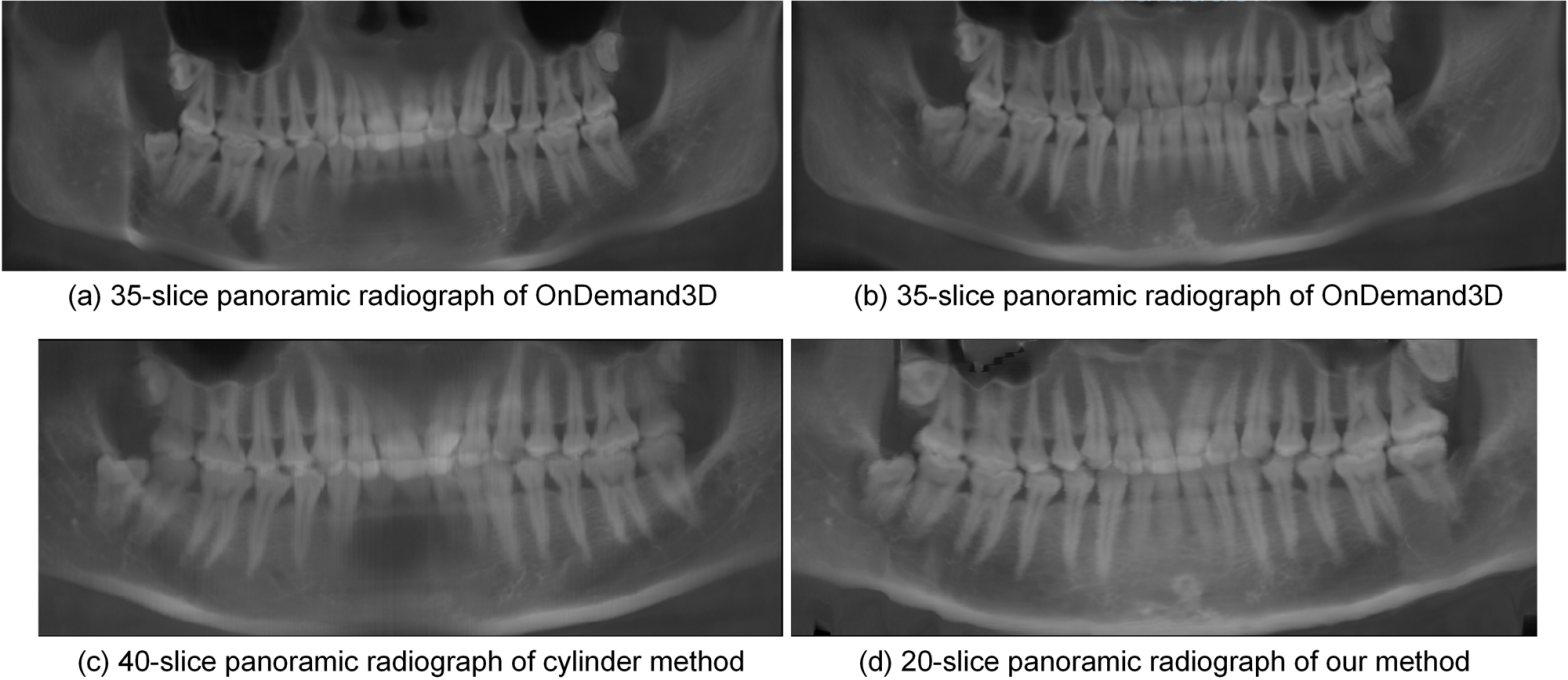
Thickened panoramic radiographs by different methods from dataset 2.
